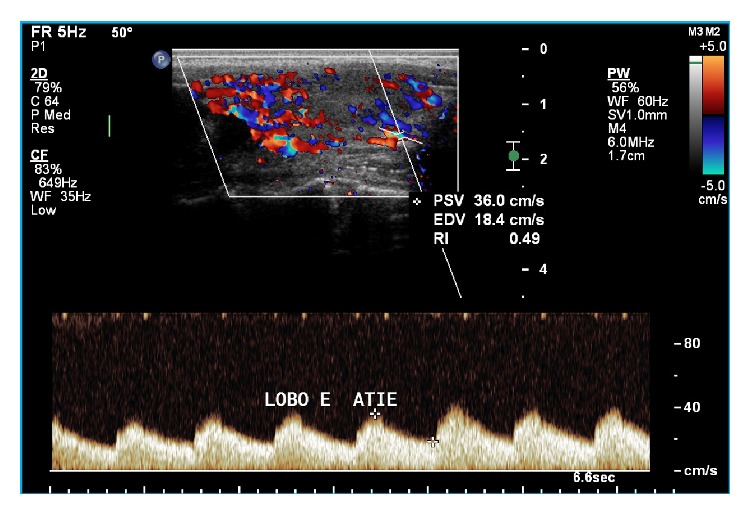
Evaluation of the PSV, EDV, and resistive index of the left inferior thyroid artery via pulsed Doppler analysis.
